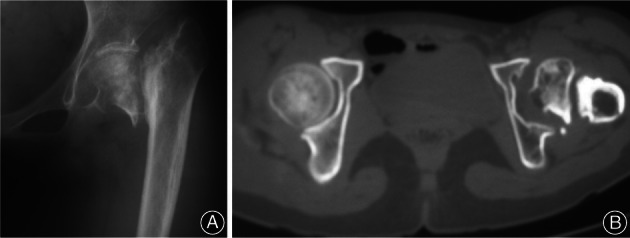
Radiographs and computed tomography images of femoral neck at the patient 16 years old shows nonunion of Garden IV femoral neck fracture.
